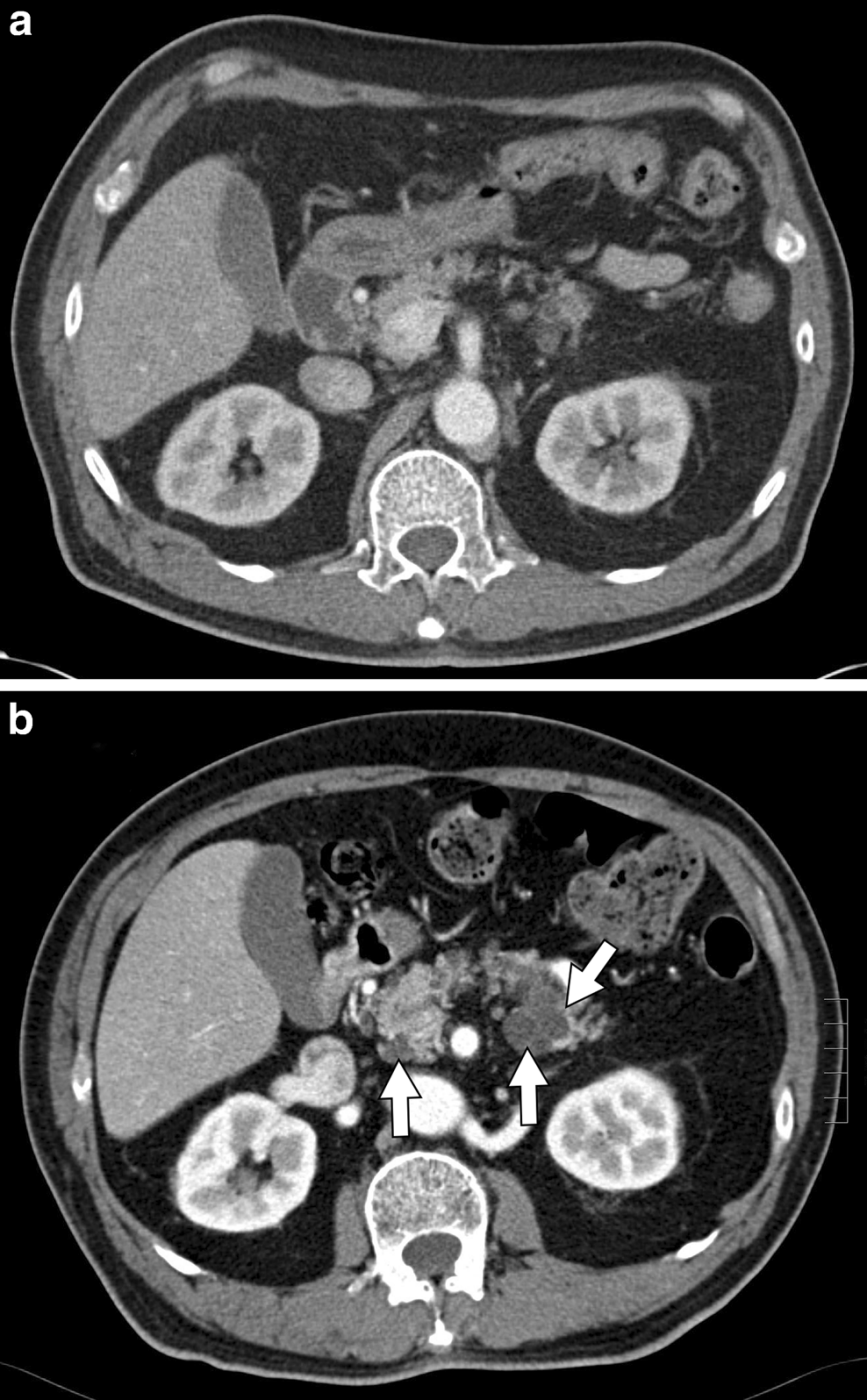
A 75-year-old male with lung adenocarcinoma receiving tarceva chemotherapy. a Axial contrast-enhanced CT image performed prior to initiation of chemotherapy treatment shows normal pancreatic features. b Axial contrast-enhanced CT performed following 2 years of chemotherapy shows the progression and development of multiple low-attenuating cystic lesions (*arrows*)
